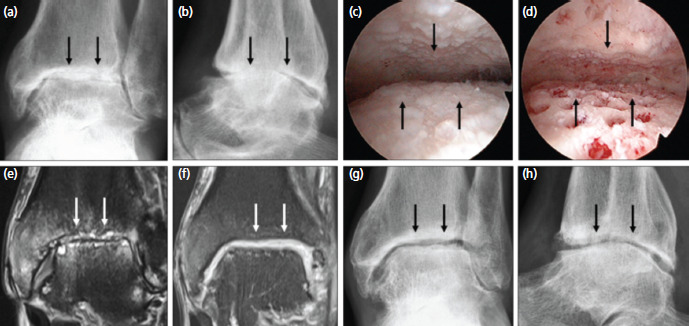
(a) Anteroposterior and (b) lateral radiographs of the left ankle joint showing end-stage arthritis (black arrows). (c) Arthroscopic view of the left ankle joint showing diffuse bone-on-bone cartilage loss (black arrows). (d) View after arthroscopic subchondral drilling of almost the entire ankle joint (black arrows). (e) Coronal MR image showing advanced arthritic changes (white arrows). (f) MR image at two years following chondrogenesis with satisfactory cartilage thickness on both articular surfaces (white arrows). (g, h) Radiographs at two years showing reappearance of the ankle joint space (black arrows). The MR images are fat-suppressed proton density-weighted.
